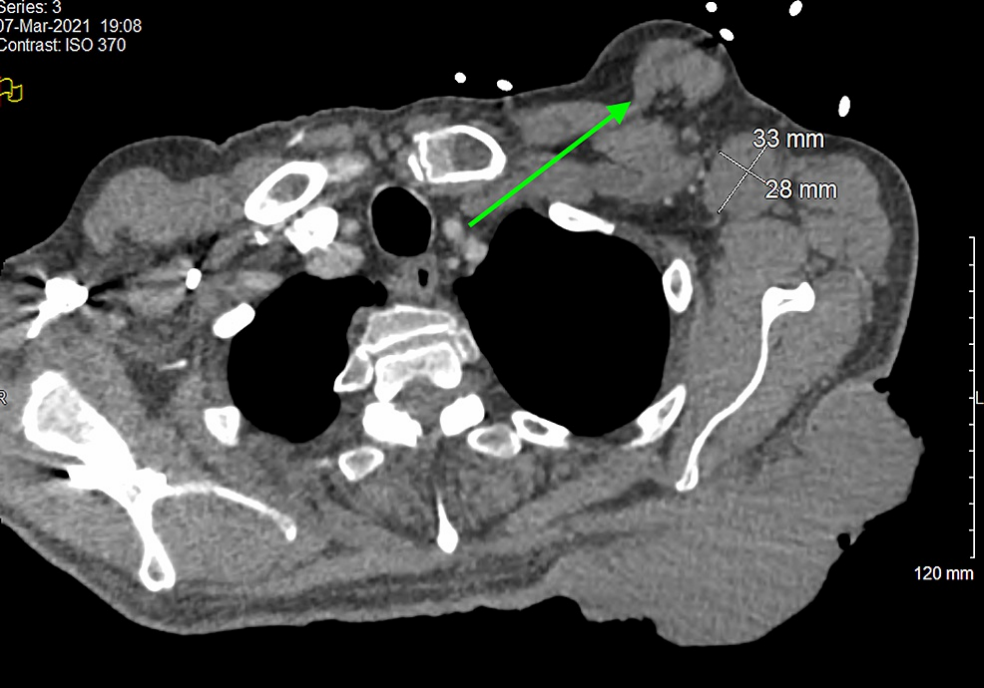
Contrast-enhanced CT image of the thorax. The mass is seen at the level of the scapula with superficial muscle compartment involvement. In addition, moderate pleural effusions and right basilar atelectasis are visualized. CT: computed tomography
